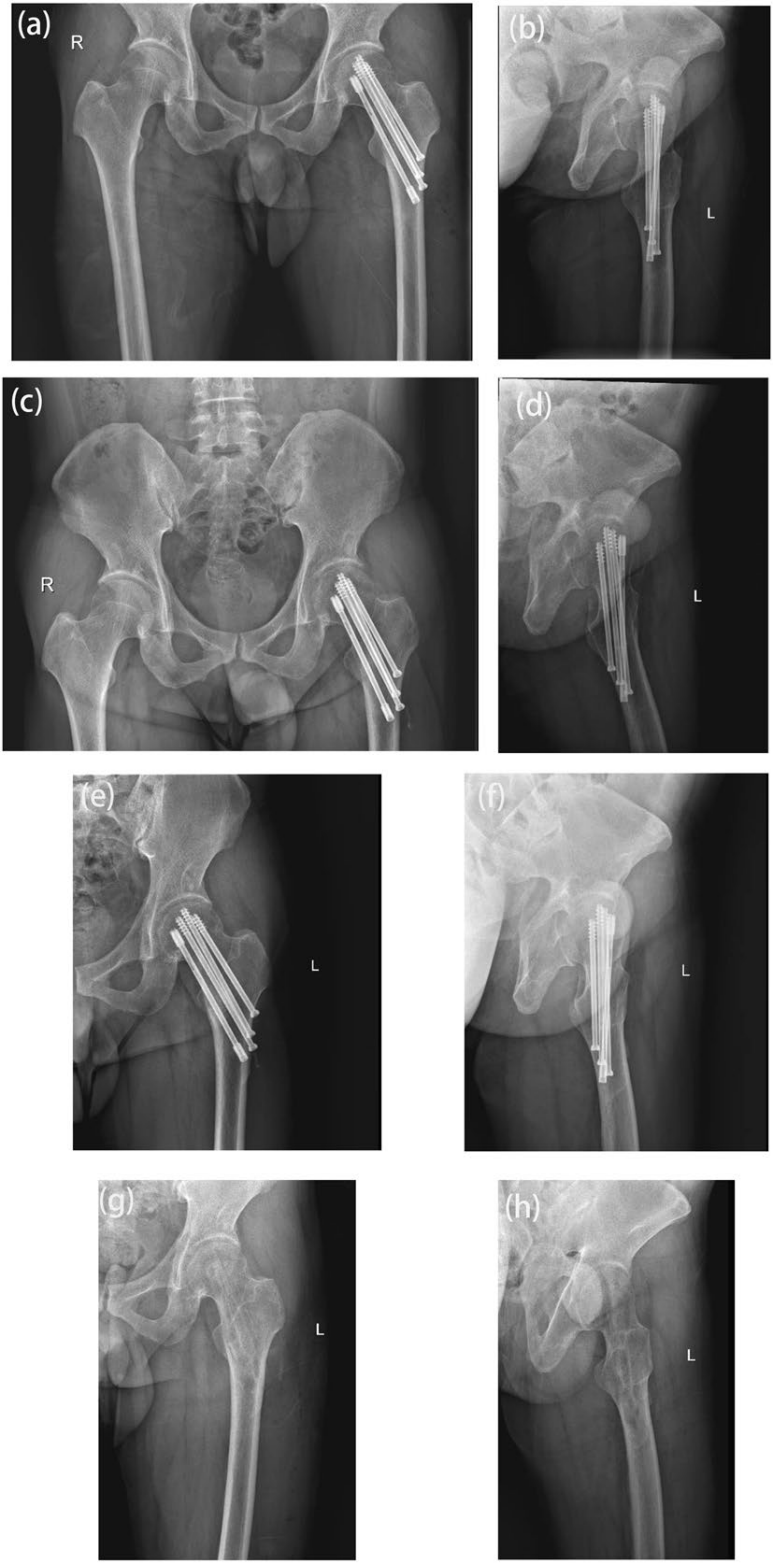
Postoperative follow-up X-ray confirming the successful healing of the left femoral neck fracture without femoral head necrosis. a–b Anteroposterior and lateral hip joint positions on the first day after surgery. c–d Anteroposterior and lateral hip joint views at 3 months post-surgery. e–f Anteroposterior and lateral hip joint positions one year after surgery. g–h Reassessment of hip joint anteroposterior and lateral positions after the removal of internal fixation
